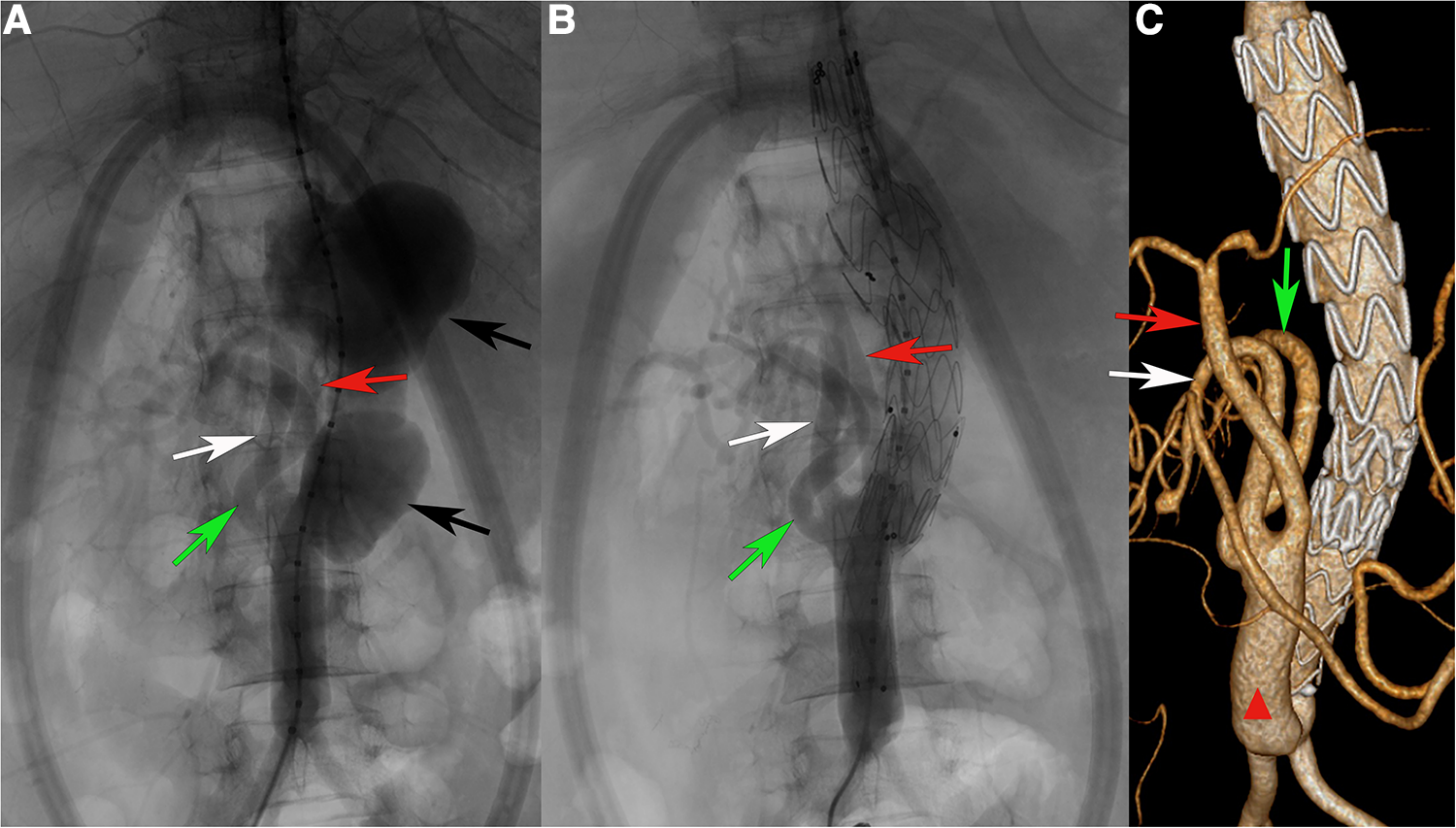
(A) Angiography after completion of splanchnic artery branch reconstruction. (B) Angiography after completion of stent placement. (C) After 5 years of follow-up, CTA showed that the pseudoaneurysm cavity completely disappeared, and the stent and branch arteries were patent. Green arrow, right renal artery. White arrow, superior mesenteric artery. Red arrow, celiac artery. Black arrow, abdominal aortic pseudoaneurysms. Red triangle: Artificial blood vessel.
